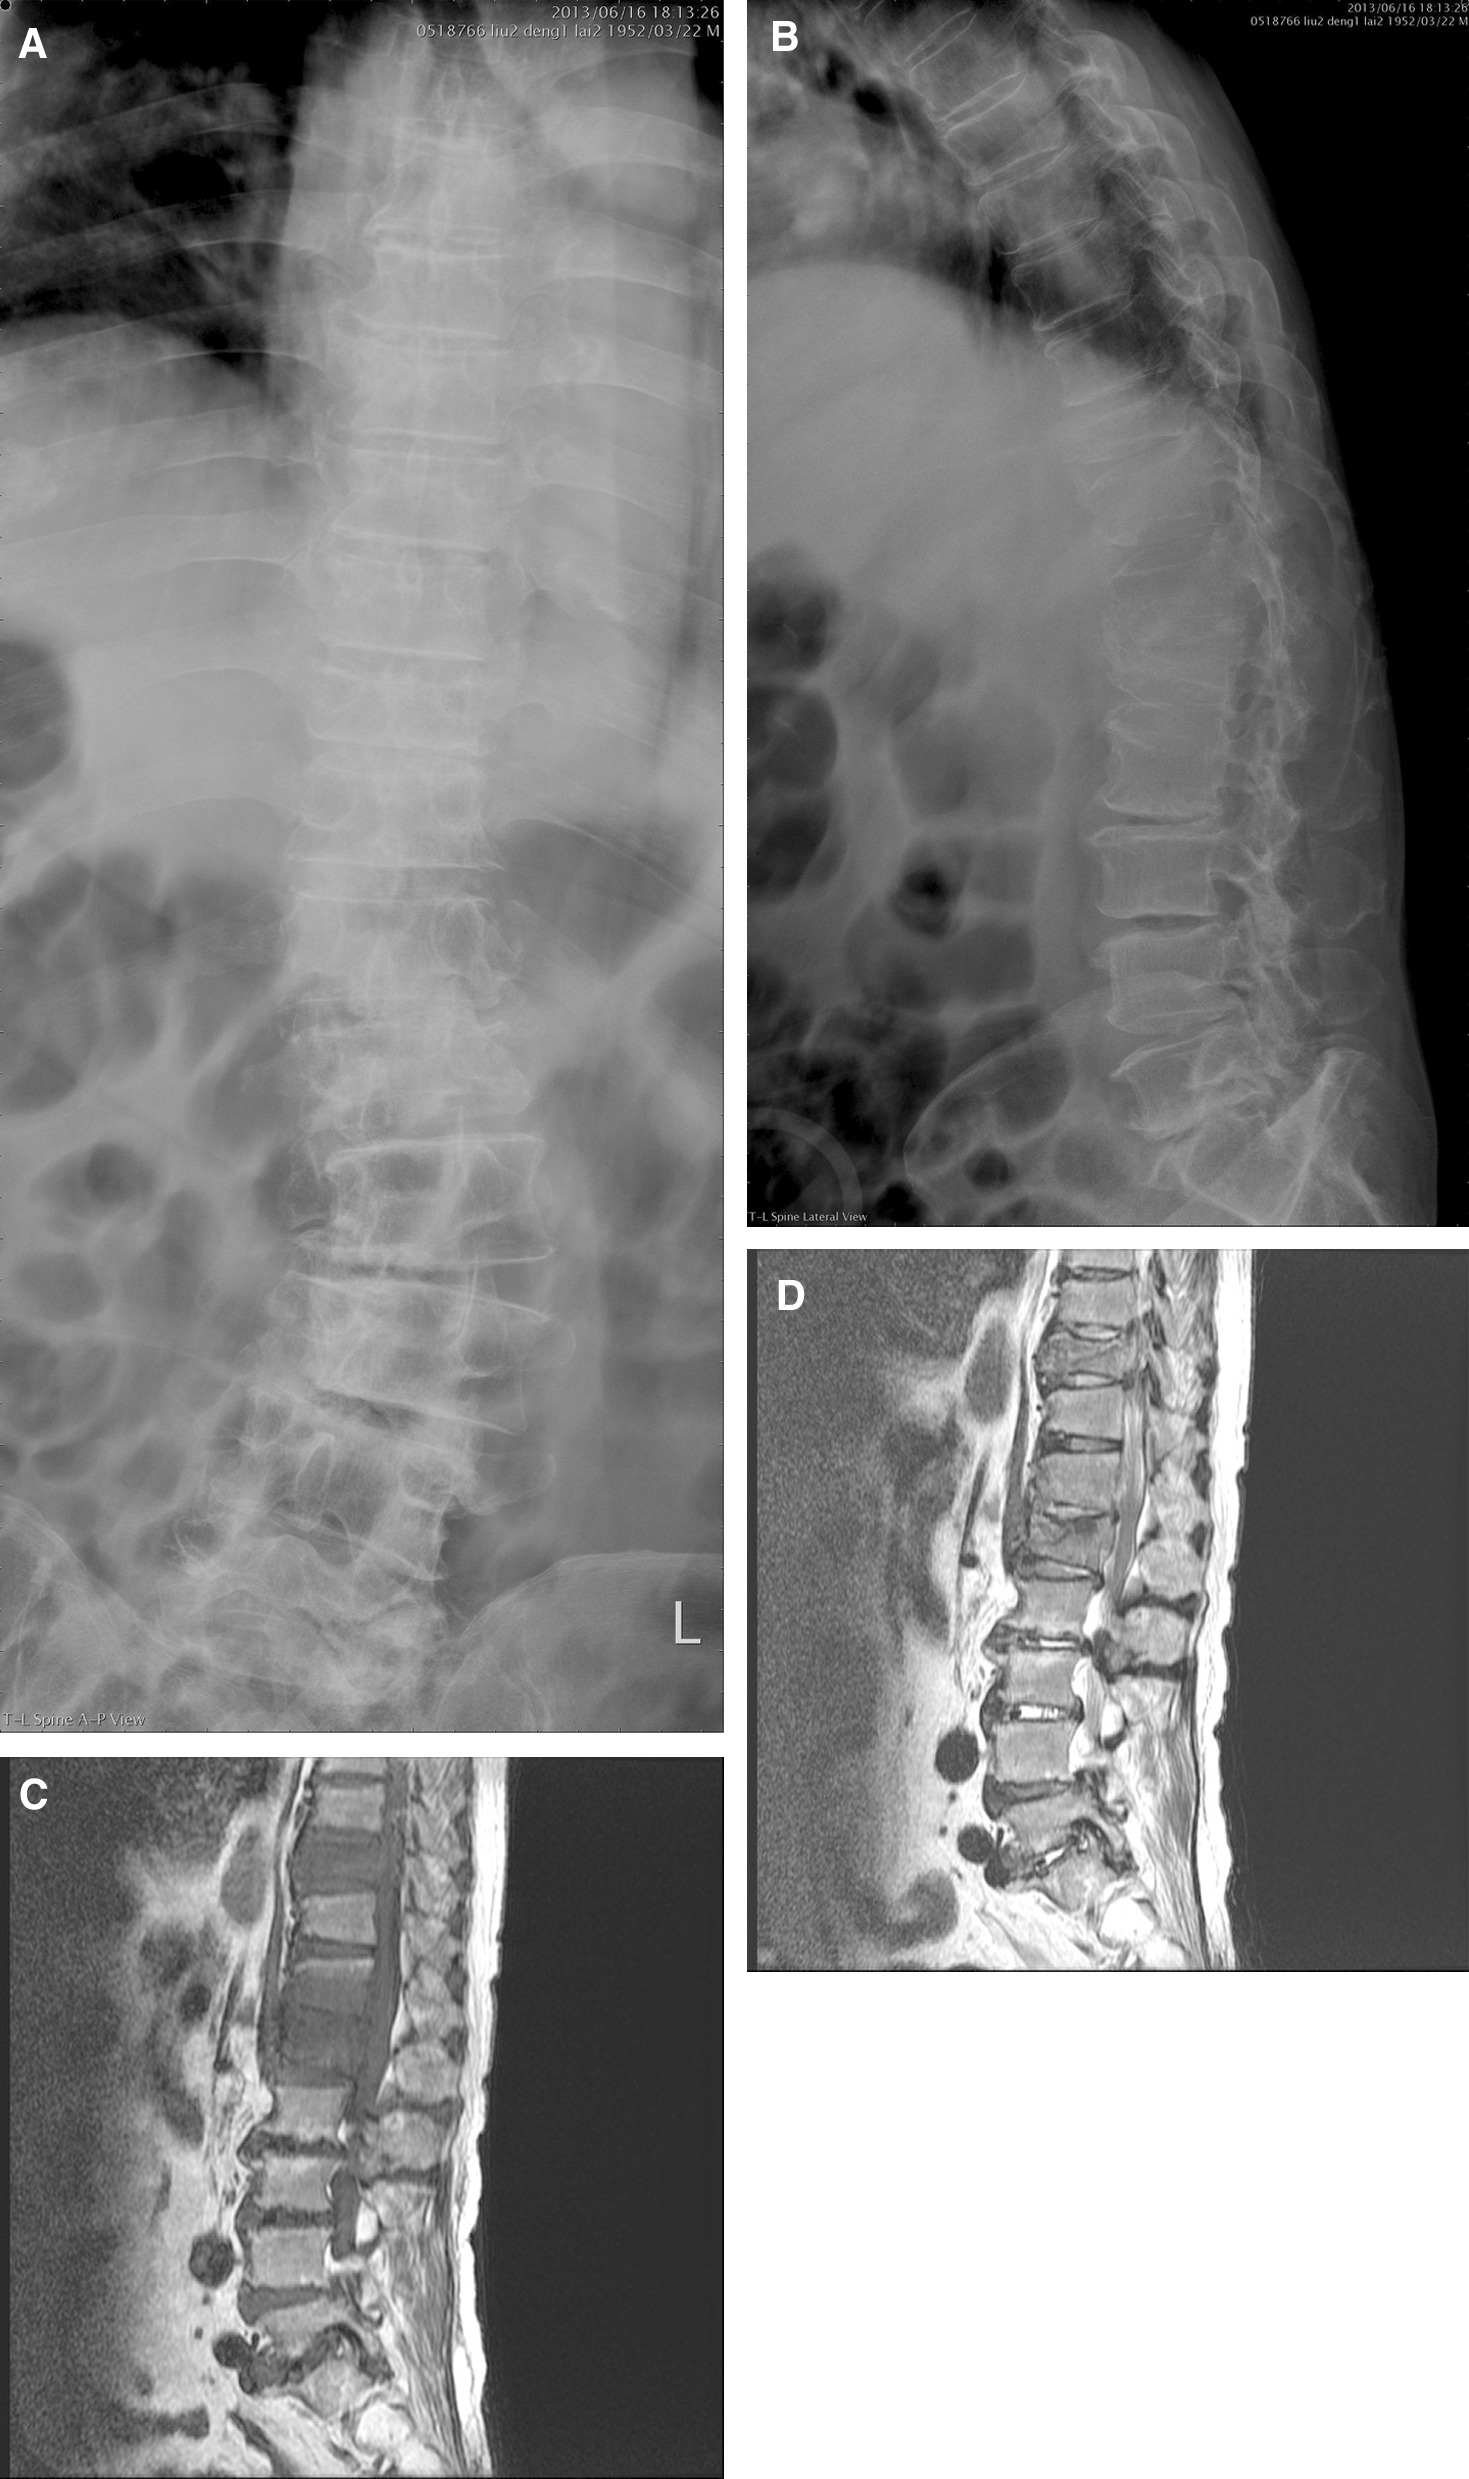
A 60-year-old male patient came to our outpatient clinic due to progressive back pain and intermittent onset of low-grade fever for more than one month. Anteroposterior (A) and lateral (B) view radiographs show vertebral body collapse of T10 and L1. T1-weighted (C) and T2-weighted MRI (D) reveals skipped spinal TB over T10 and L1 levels
